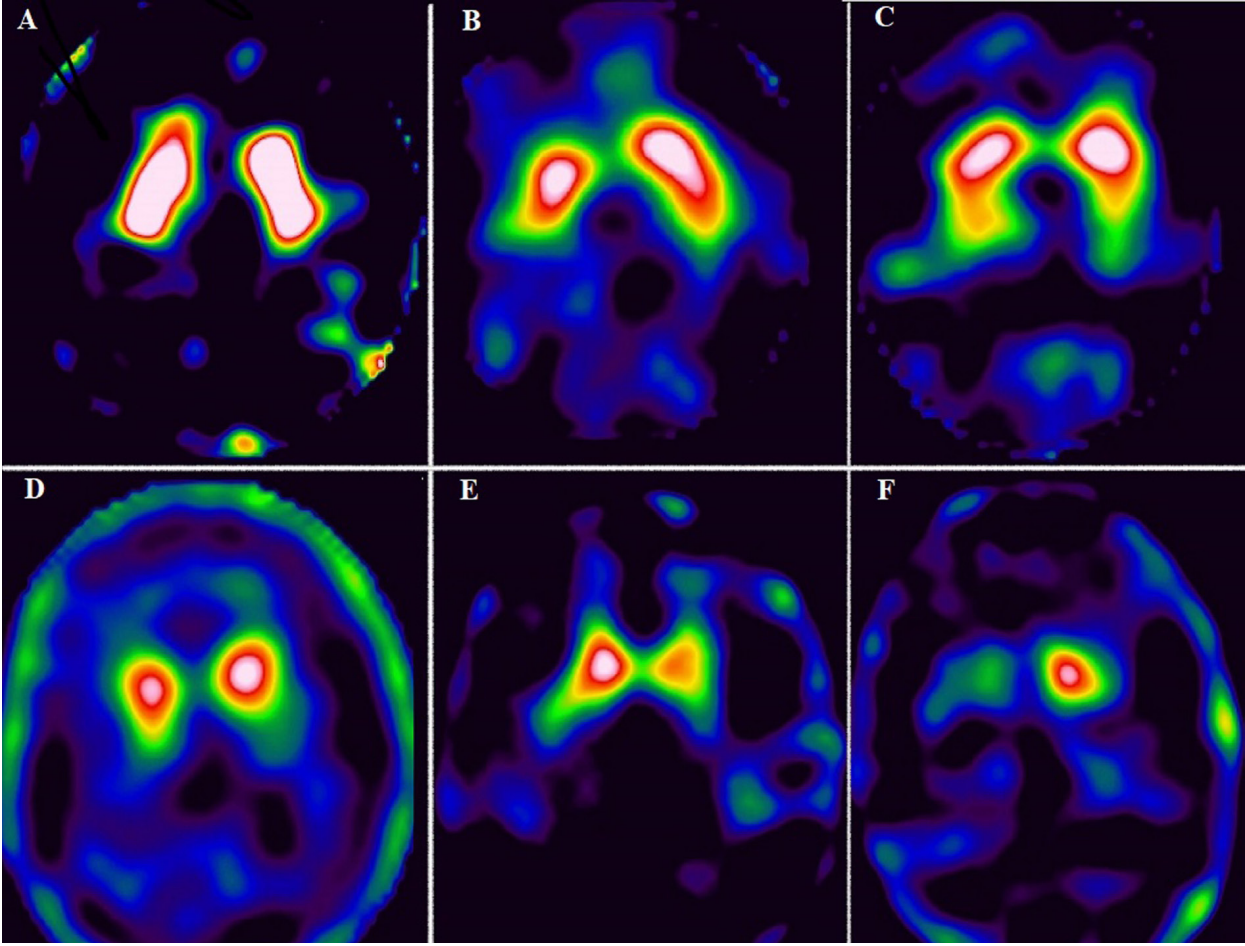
Tc-99m TRODAT-1 SPECT image of control (A) and patients belonging to different H and Y stages (B-F). B: stage 1, patient had history of rest tremors in left upper limb since 2 years; image shows reduced uptake in right putamen; C: stage 2, patient presented with difficulty in writing, rest tremor in bilateral upper limb, more on right side, image shows reduced ^99^^m^Tc-TRODAT-1 uptake in bilateral putamen (L<R) with preserved uptake in bilateral caudate nuclei; D: stage 3, patient had bilateral rest tremor and difficulty in walking; image shows no significant uptake in bilateral putamen, reduced uptake in right caudate nucleus. Uptake in left caudate nucleus is preserved; E: stage 4, patient presented with slowness of movements, rest tremors, difficulty in walking without support; image shows no significant uptake in bilateral putamen and reduced uptake in left caudate nucleus. Uptake in right caudate nucleus also appears reduced; F: stage 5, patient had rest tremors, was wheel chair bound, rigidity in all 4 limbs (R>L); image shows no significant uptake in bilateral putamen and right caudate nucleus, and reduced uptake in left caudate nucleus
